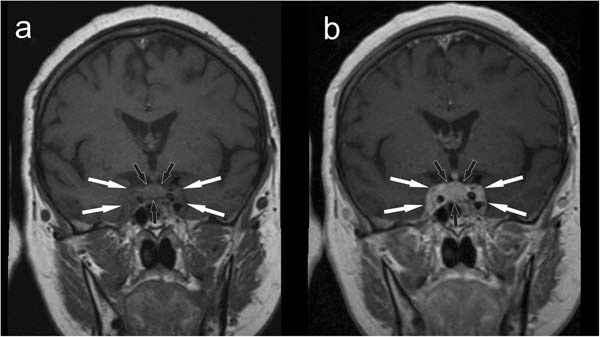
Initial MRI scan, coronal plane, before (a) and after (b) contrast medium administration. The pathological infiltrate involving the pituitary is marked with black arrows. White arrows mark the extent of the infiltrate, which involves both cavernous sinuses
